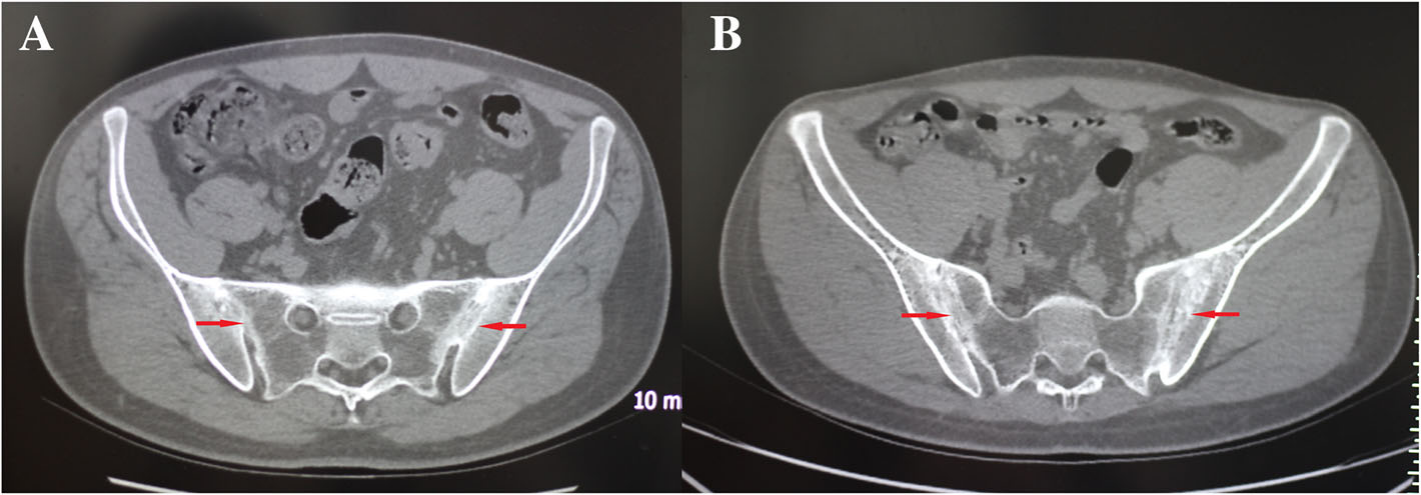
Radiograph of two representative ankylosis spondylitis patients with bilateral grade 4 sacroiliitis: a male, 28 years old; b male, 25 years old
